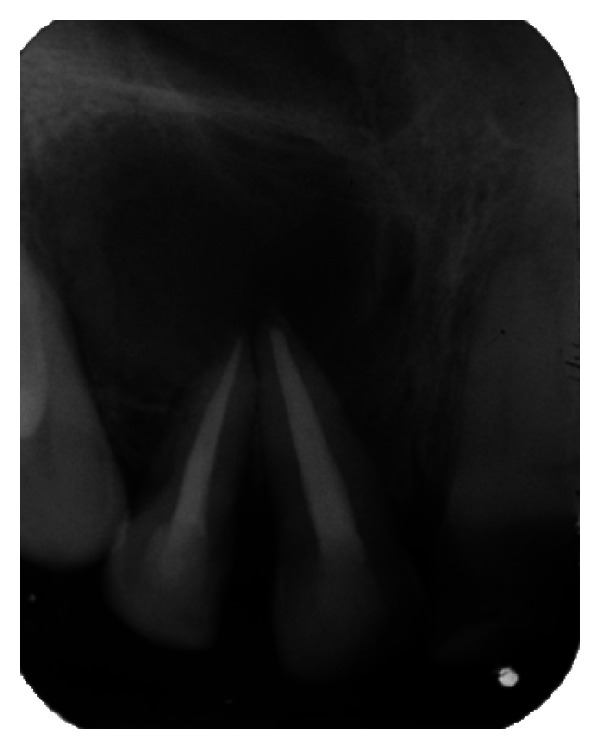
Postobturation IOPA radiograph.
